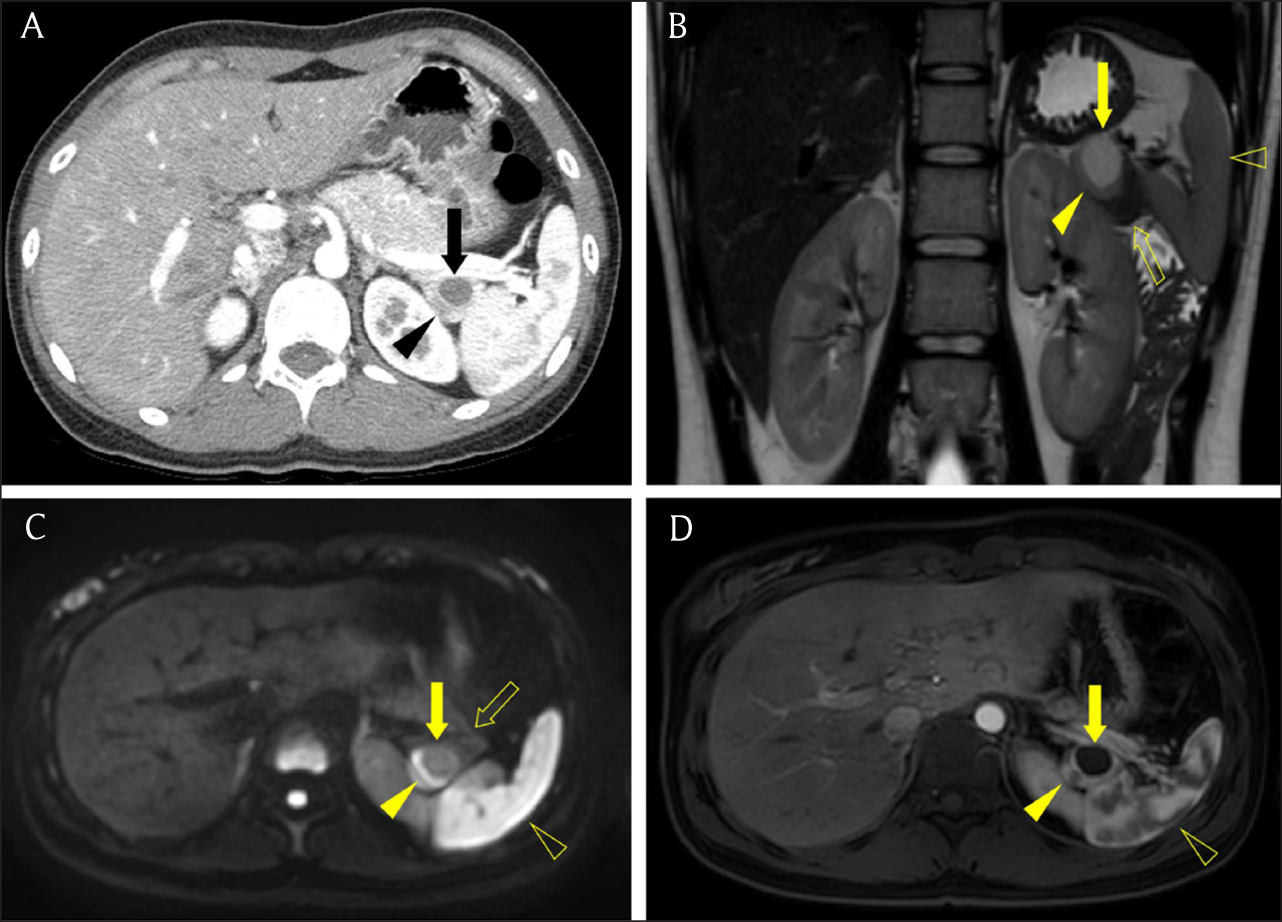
A 22-year-old woman with epithelial cyst arising from intrapancreatic accessory spleen. (A) Axial pancreatic phase CT image shows a 2.5 cm cystic lesion (arrow) with solid component (arrowhead) in the pancreatic tail. (B, C) MR images show that the signal intensity of the solid component (arrowhead) on T2-weighted image (B) and diffusion-weighted image (C) is closely similar to that of the spleen (open arrowhead) and different from that of the pancreatic tissue (open arrow). (D) Axial gadolinium-enhanced arterial phase MR image reveals zebra enhancement pattern of the solid component (arrowhead), like as spleen (open arrowhead). Note cystic portion (arrow) without enhancement.
